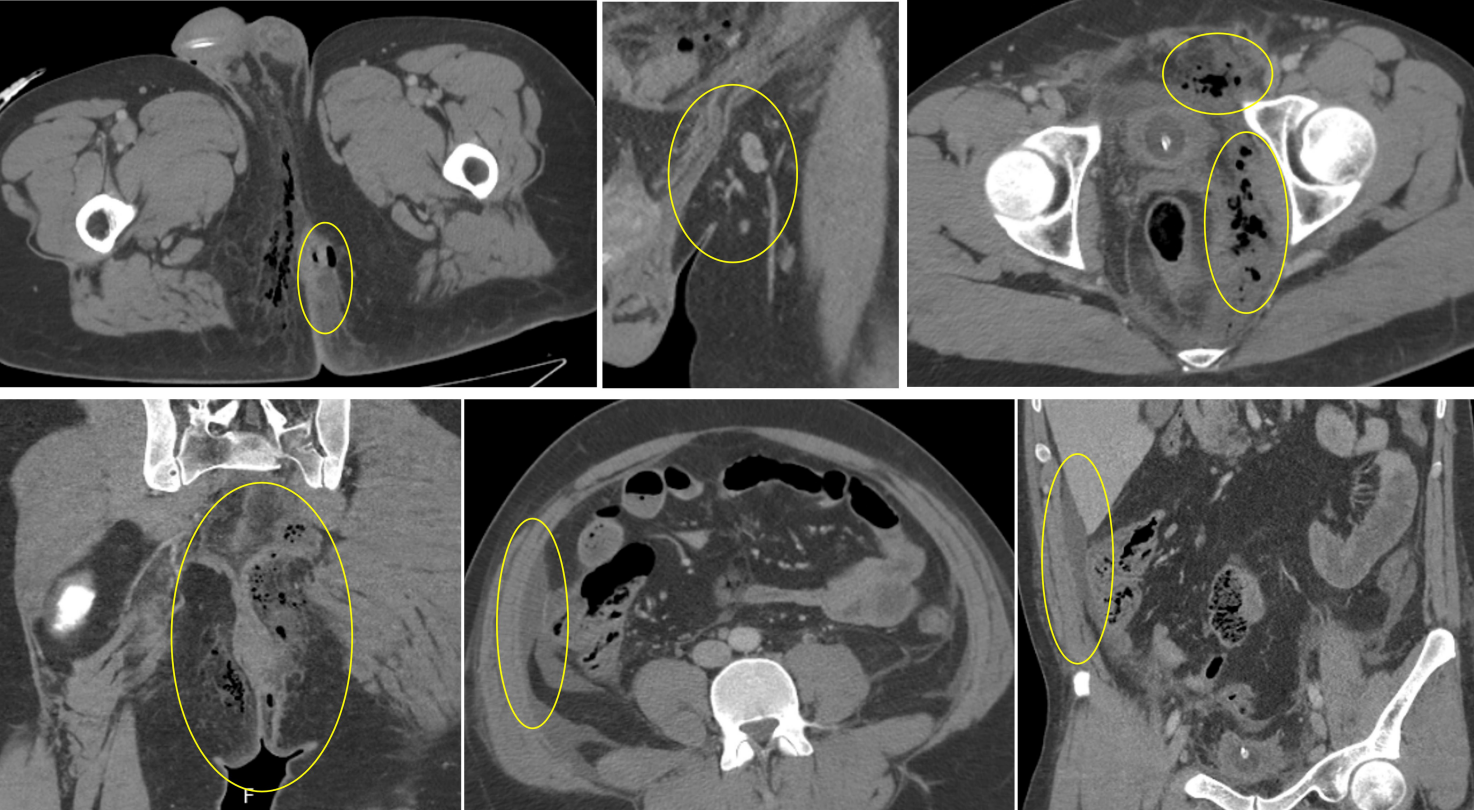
Abdominal CT scan shows a 6.3 × 2.6 × 4.9 cm^3^ left perianal abscess with gas accumulation in the space of Retzius, base of the penis, intergluteal region, and ischiorectal fossa. Additionally, there was free fluid in the pelvic region and along the parietocolic gutters, as well as enlarged lymph nodes in the left external iliac region measuring approximately 15 mm in short‐axis diameter. Findings consistent with extensive necrotizing fasciitis, specifically Fournier's gangrene.
